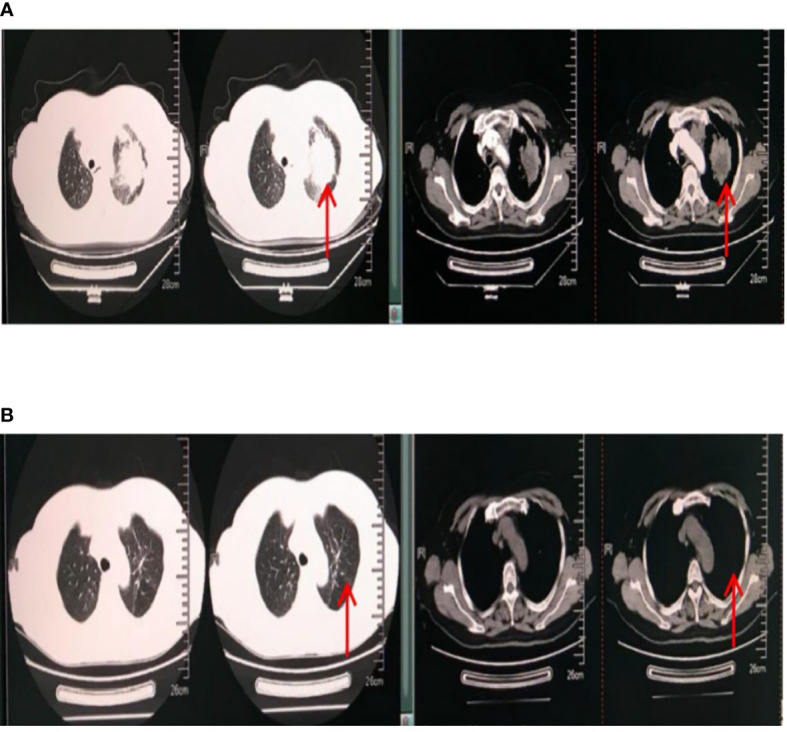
Chest CT results. The red arrow points to the lung tumor. (A) Chest CT results on 13 December 2017 (before treatment). The maximum cross-sectional area of the space-occupying lesion in the upper lobe of the left lung was 7.3 cm × 5.5 cm. Multiple metastatic lymph nodes were found in the mediastinum and neck. There was pleural effusion in the left chest. (B) Chest CT results on 27 May 2019 (after treatment). The maximum cross-sectional area of the space-occupying lesion was 9 mm × 4 mm. Multiple small lymph nodes were found in the mediastinum and neck.
